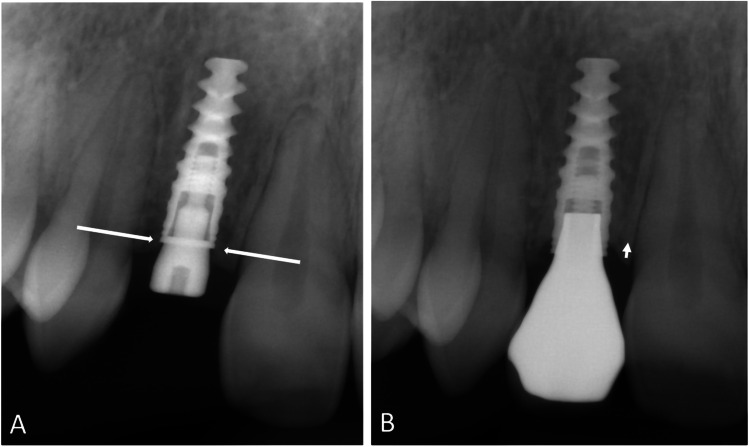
Measurement of marginal bone level related to the level of the implant shoulder mesial and distal to the implant (marked with arrows) on intra-oral radiographs exposed perpendicular to the implant shoulder. A Examination at baseline; the bone level exceeded the platform of the implant and were scored as 0. B Examination after 1 year of loading
